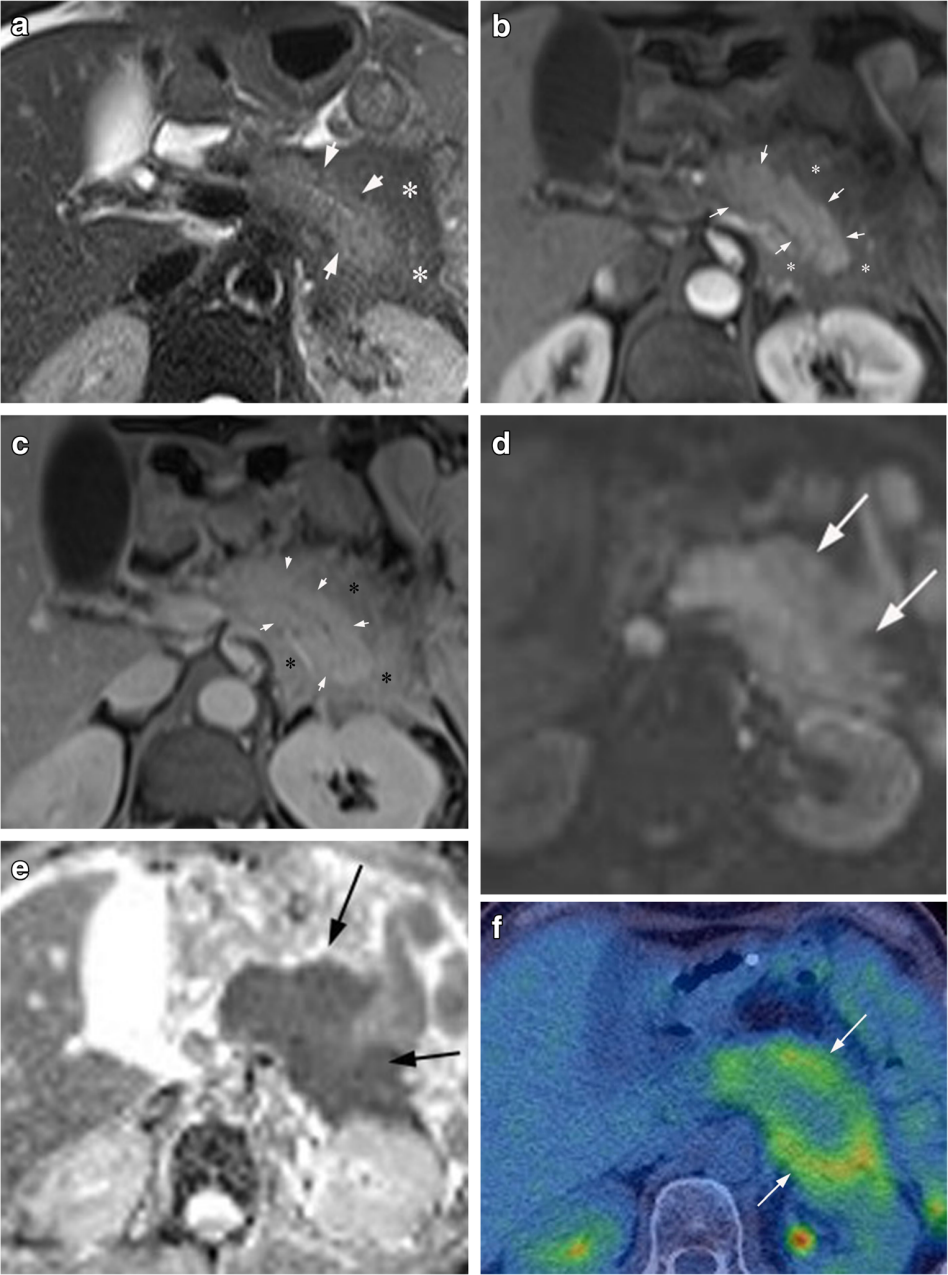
A patient with a history of mucosa-associated lymphoid tissue (MALT) lymphoma, presenting with raised serum IgG4 and diagnosis of AIP on endoscopic ultrasound (EUS)-guided biopsy. a Axial T2 fat saturated (FS) turbospin echo image shows intermediate to high T2 signal within the pancreas (*arrows*), but extensive low T2 signal peripancreatic tissue (*). There is diffuse narrowing of the distal pancreatic duct. b Axial T1FS post-contrast arterial phase initially shows reduced enhancement of the peripancreatic tissue (*) compared to the pancreas (*arrows*). c Axial T1FS post-contrast equilibrium phase showing delayed enhancement of the peripancreatic tissue. d The pancreas and extrapancreatic soft tissue shows marked restricted diffusion with high signal on diffusion-weighted imaging—B800 (*arrows*), a typical finding in AIP. e The tissue demonstrates corresponding low signal on the ADC map (*arrows*). f Only the extrapancreatic soft tissue shows high-grade FDG uptake (*arrows*), a finding which was felt to be atypical for lymphoma
